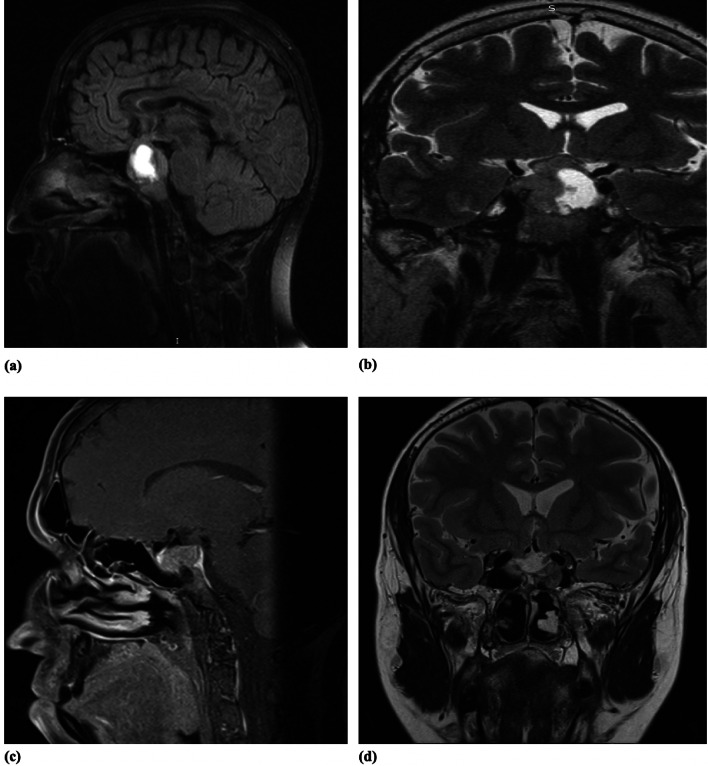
MRI coronal and sagital images. a – MRI at the time of diagnosis – hyperintense area at the left side of the pituitary gland of 52/38/52 mm. b – Postoperative MRI – no significant regression of the lesion – c, d – control MRI after radiotherapy and medical treatment
